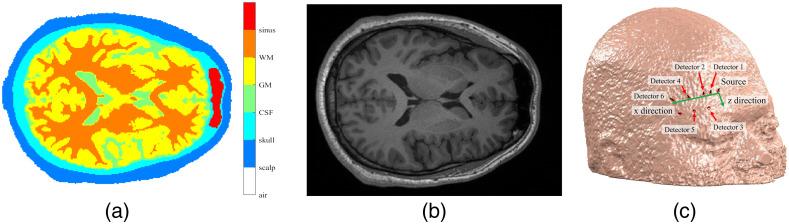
The head model (a) a slice of the segmented head model, (b) the corresponding MRI scan, and (c) positions of the source and detector fibers at the head surface.
